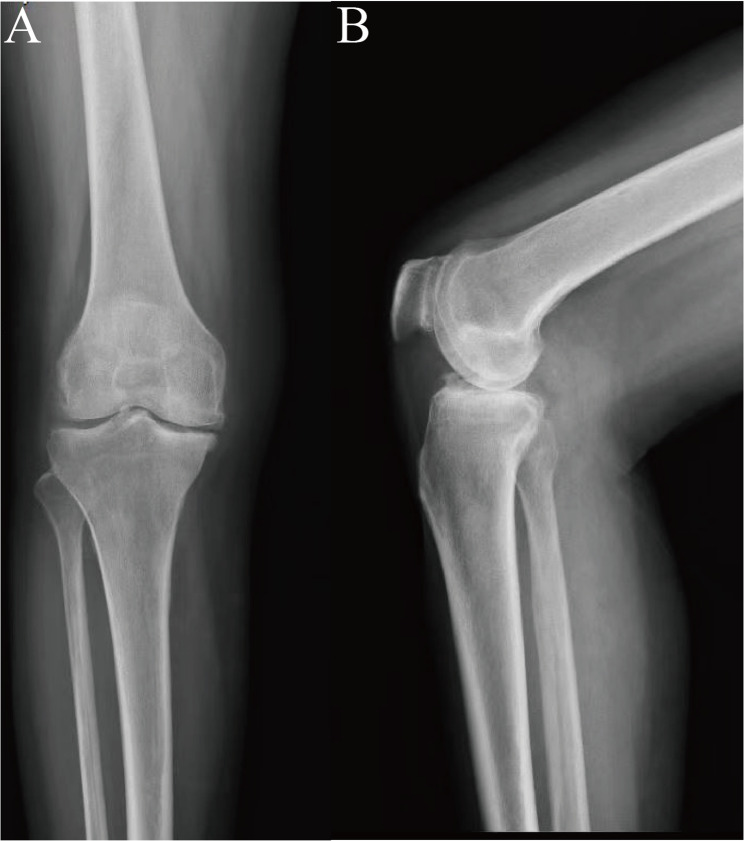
Anteroposterior and lateral radiographs of the knee before unicompartmental knee arthroplasty (A and B). Narrowing of the knee joint space and sclerosis of the articular surfaces (A and B)
